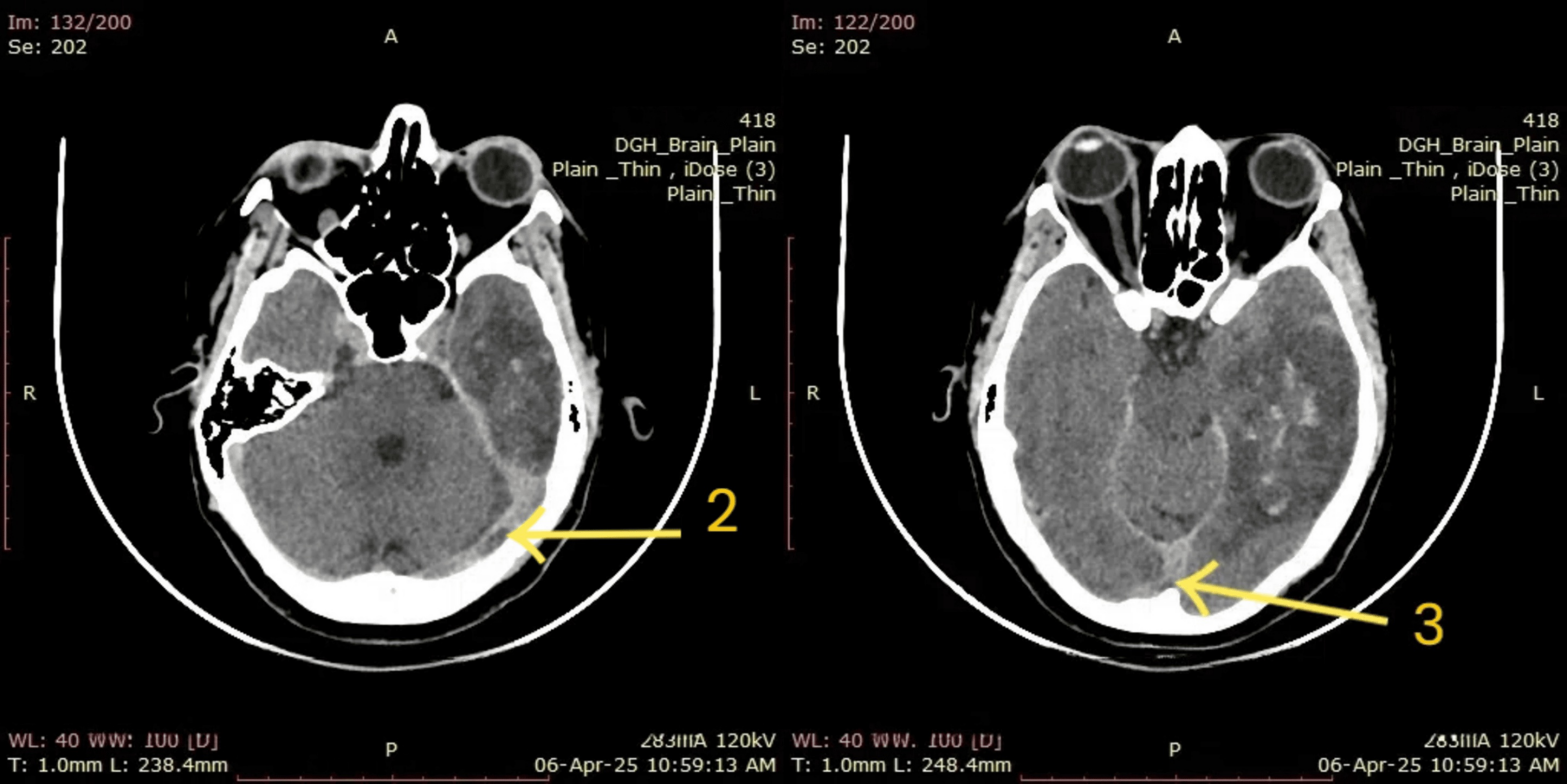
CT venogram - brain Arrow 2: There is a clear filling defect within the left transverse sinus, demonstrating a lack of opacification in comparison with the contralateral side. Arrow 3: Confluence of sinuses shows a poor contrast filling, consistent with propagation of thrombus from the transverse.
